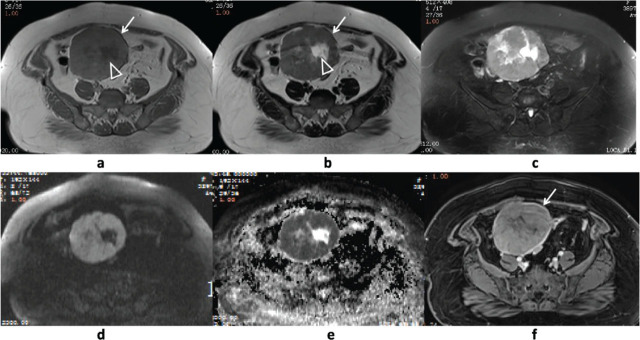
Pelvic MRI revealed a solid oval mass in the right adnexal region. It exhibited iso-signal intensity on T_1_WI (a), slightly higher-signal intensityonT_2_WI (b, relative to muscle), and T_2_WI fat-suppressed axial images (c). The mass possessed a capsule (indicated by the arrow in a,b) and central cystic necrosis (triangular arrow in a,b). The mass displayed marked high intensity on the diffusion-weighted image (B = 800 s/mm^2^) (d) with restricted diffusion on the ADC map (e). The solid part of the mass exhibited homogeneous and moderate enhancement with intravenous gadolinium-DTPA, and the envelope (arrow) also demonstrated enhancement (f). ADC, apparent diffusion coefficient; MRI, magnetic resonance imaging.
